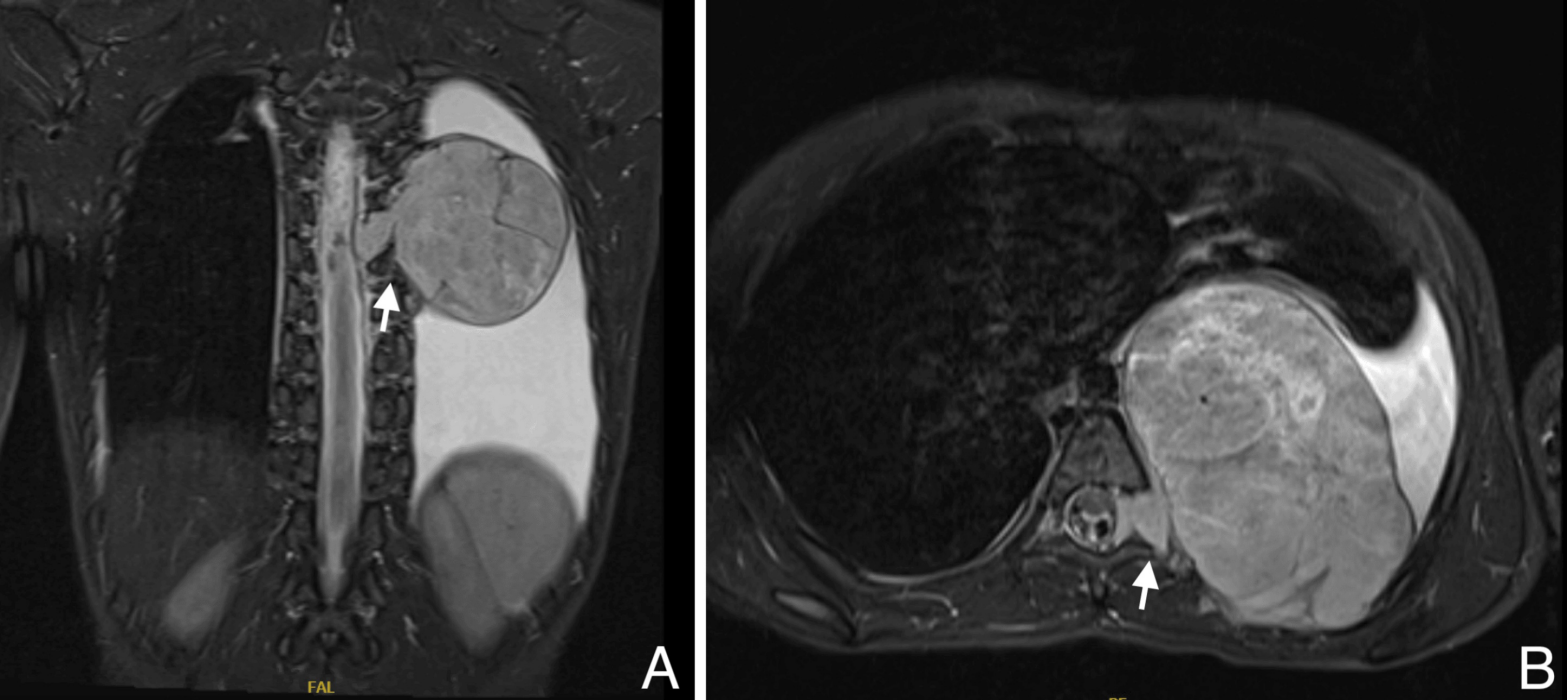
T2 MRI of the chest Preoperative T2 image MRI of the chest, coronal (A), and axial (B) views showing a 50x76x62 mm lesion within the posterior mediastinum extending through the left neural foramina of T6 that caused right-sided spinal deviation without spinal cord compression.
